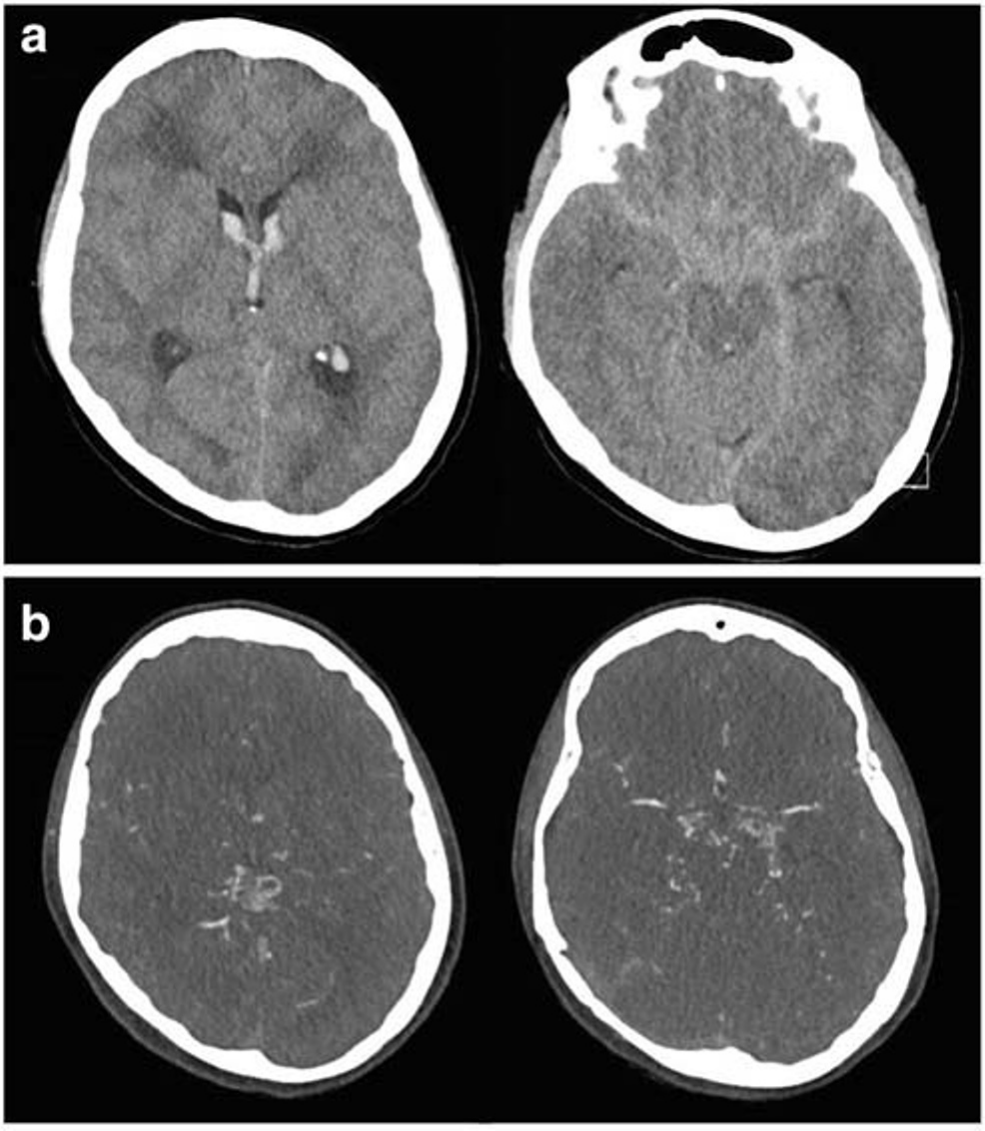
a The initial CT scan demonstrating ICH in the right hypothalamus with IVH and SAH in the basal cisterns, scored as Hunt and Hess 3/Fisher 4. Additionally, signs of diffuse leukoencephalopathy in both hemispheres with spotty calcification were observed. b The CT angiogram demonstrated stenosis of both distal internal carotid arteries (ICA) and a proximally dilated posterior communicating artery, which appeared occluded after the P1 segment. The middle (MCA) and anterior cerebral arteries (ACA) were partially fed by collaterals with a rete of collateral moyamoya vessels
